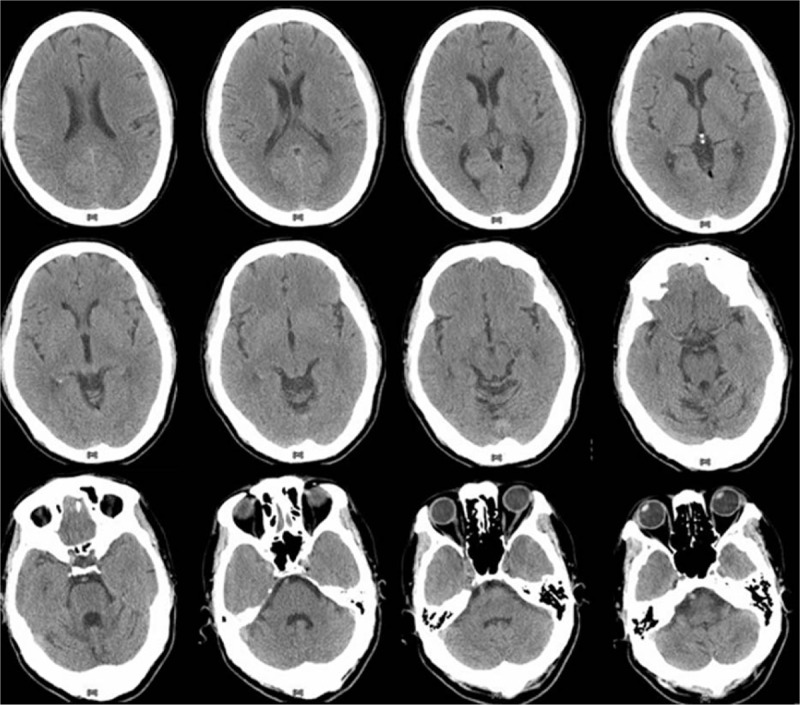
Brain computed tomography scan showed only some low attenuations in right cerebellum, favoring prior sequela lesion.
